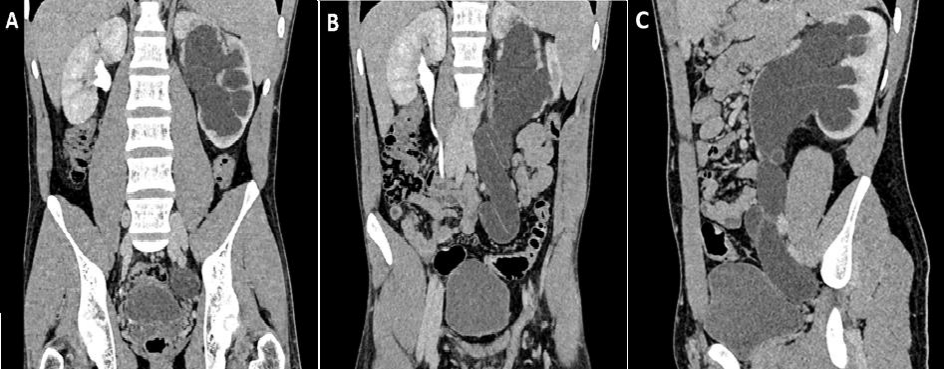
left primary obstructive megaureter: A) coronal reconstruction of the CT scan showing a left giant hydronephrosis, with marked reduction of the cortical thickness and dilation of the terminal segment of the ureter just before the ureterovesical junction; B) coronal reconstruction of the CT scan showing a left giant hydroureteronephrosis, with the absence of urinary excretion of contrast agent and huge dilatation of left ureter with tortuosity down to the urinary bladder; C) sagittal reconstruction of the CT scan showing an enlarged left kidney, with giant pelvis and ureter just before the ureterovesical junction
